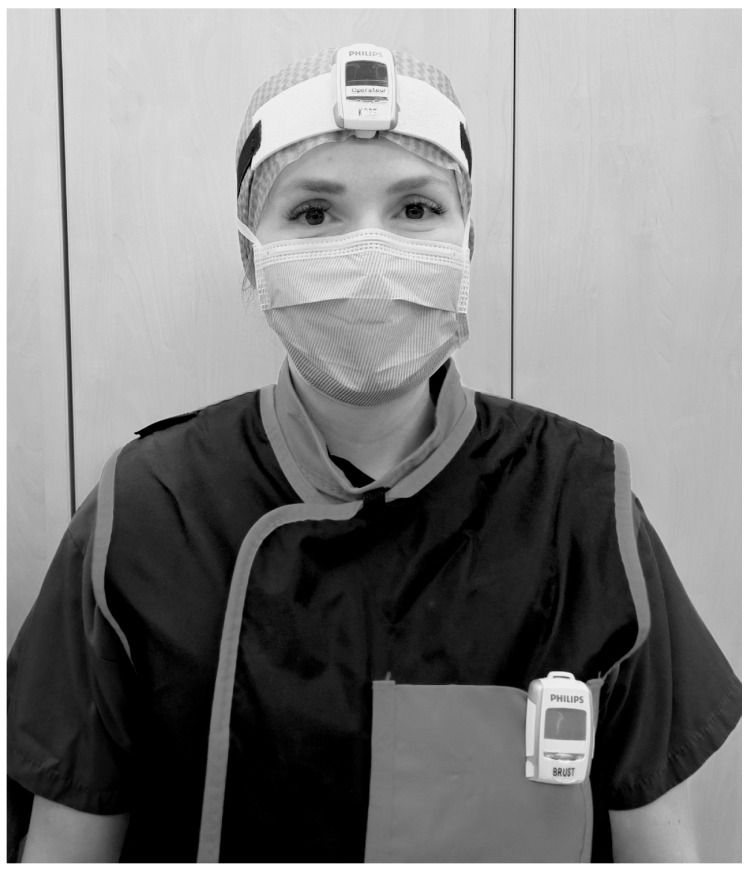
Method of wearing of the real-time dosimeters on the head (between the eyes on the forehead attached to a headband or the surgical cap) and chest (on the left chest above the X-ray apron).
